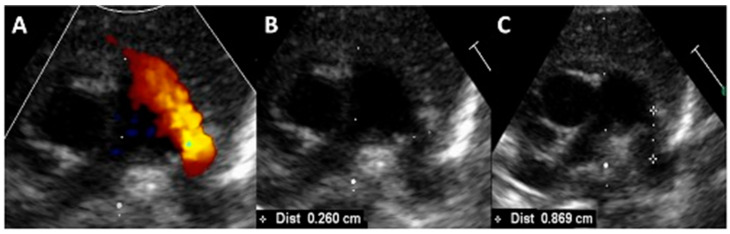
Last minute, pre-procedural echocardiography showing a large, left-to-right shunting, patent ductus arteriosus in an ex-24-week premature infant, with a procedural weight of 740 g (A). The minimal ductal diameter was measured at 2.6 mm (B), with a ductal length of 8.7 mm (C).
